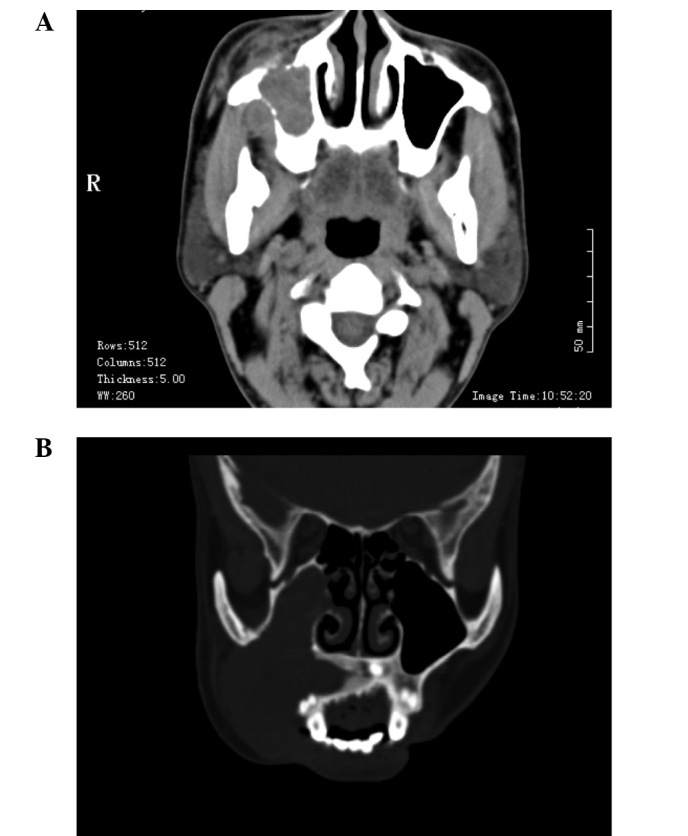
Computed tomography images of the basaloid squamous cell carcinoma. (A and B) The mass invaded the maxillary sinus and the central region of the hard palate.
